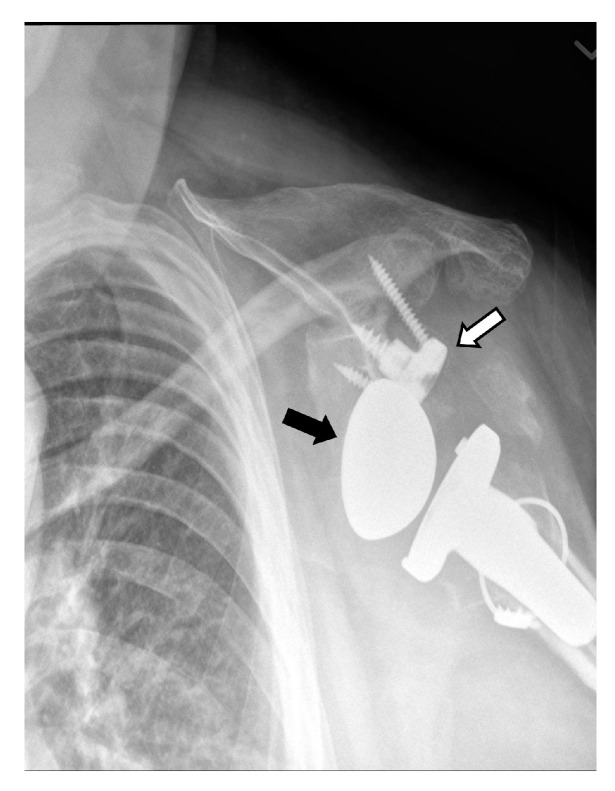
Frontal radiograph in a patient with RTSA shows dissociation of the metaglene/baseplate (white arrow) and the glenosphere (black arrow).
